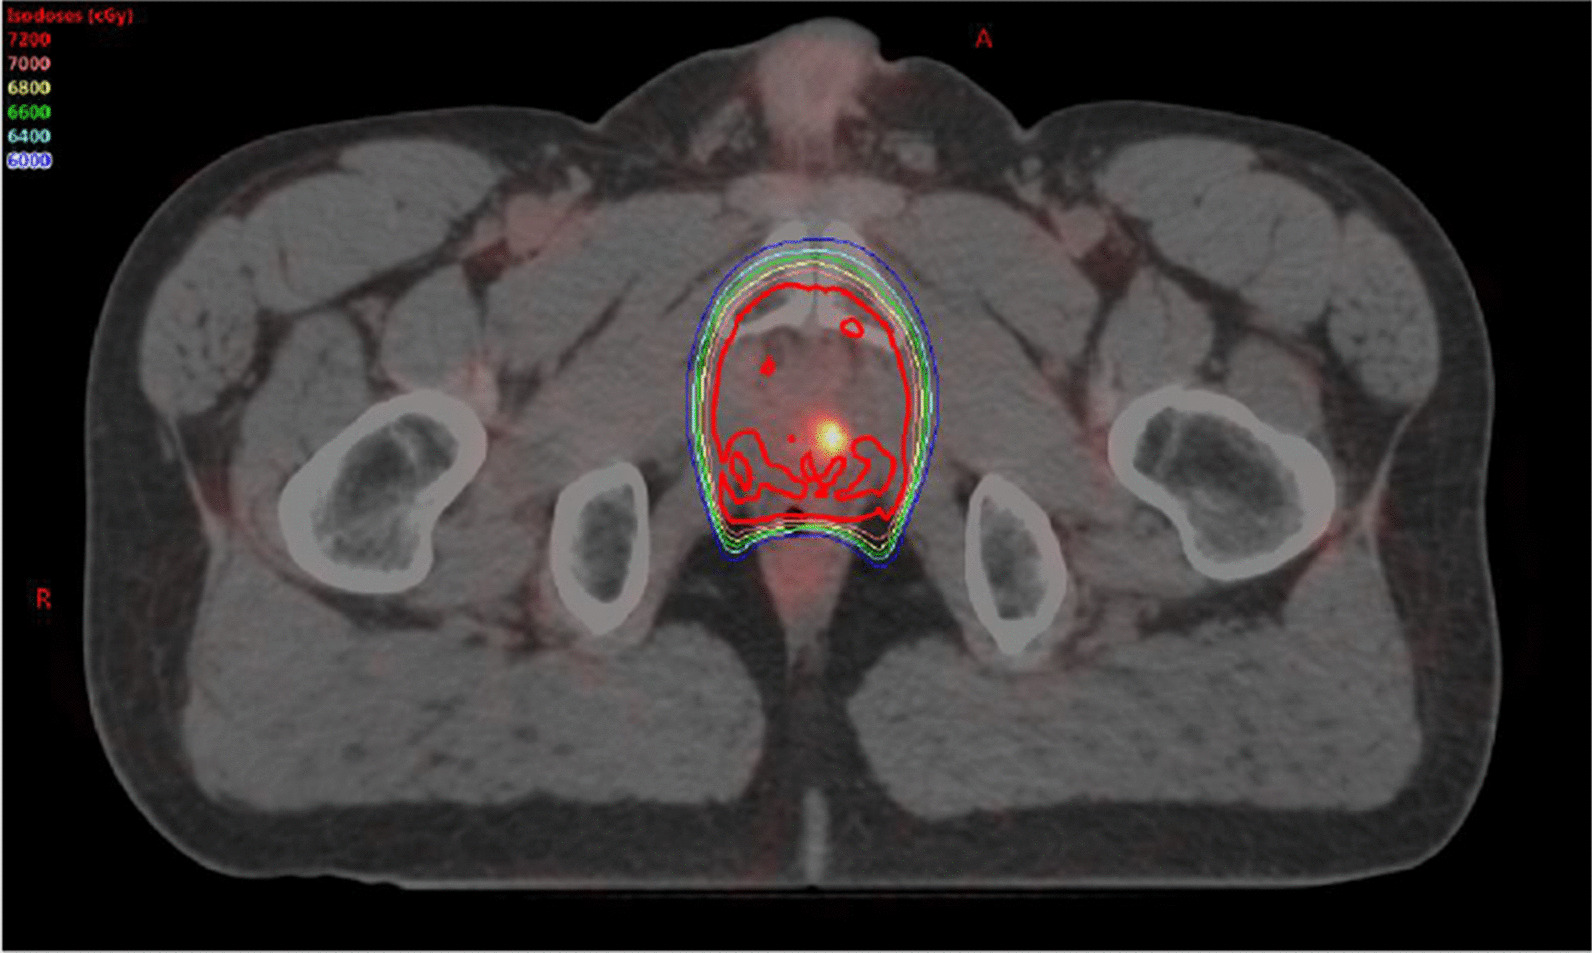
58-year-old male with a serum PSA of 3.09 ng/ml after radical prostatectomy and salvage radiotherapy with ADT. ^18^F-PSMA-PET image overlaid with radiation treatment dosimetry. The patient received a prescribed dose of 70.2 Gy
